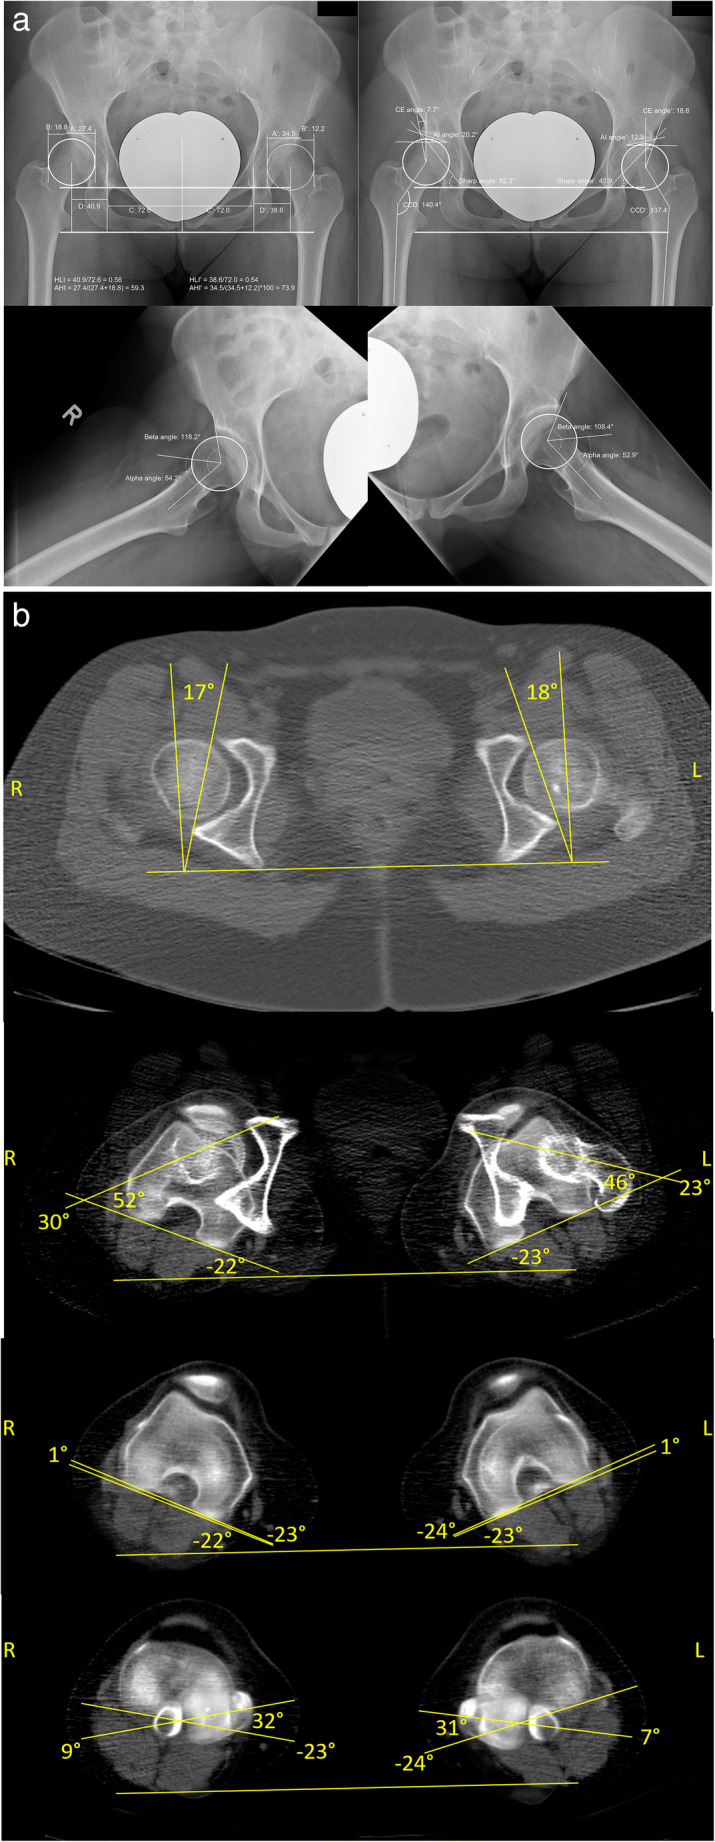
Radiographic Findings in a *dysplastic hip patient*
